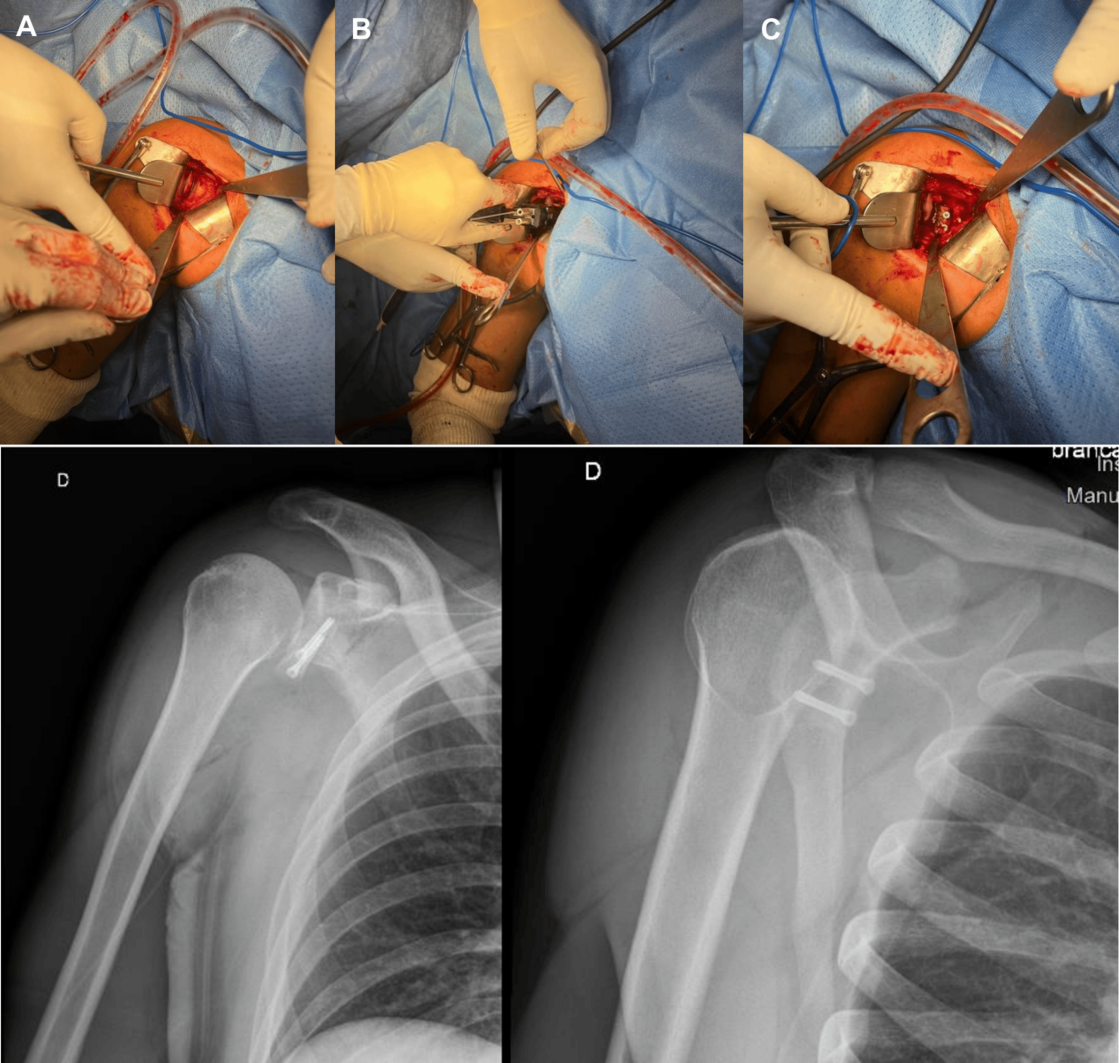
Graft placement with the HyLa instrumentation and postoperative radiographs. Final positioning and fixation of the iliac crest graft using the HyLa surgical set, with postoperative radiographs confirming proper graft alignment and fixation. (A) Exposure of the anteroinferior glenoid rim. (B. Positioning of the bone-block instrumentation at the anteroinferior glenoid rim. (C, D) Fixation of the graft with two screws.
